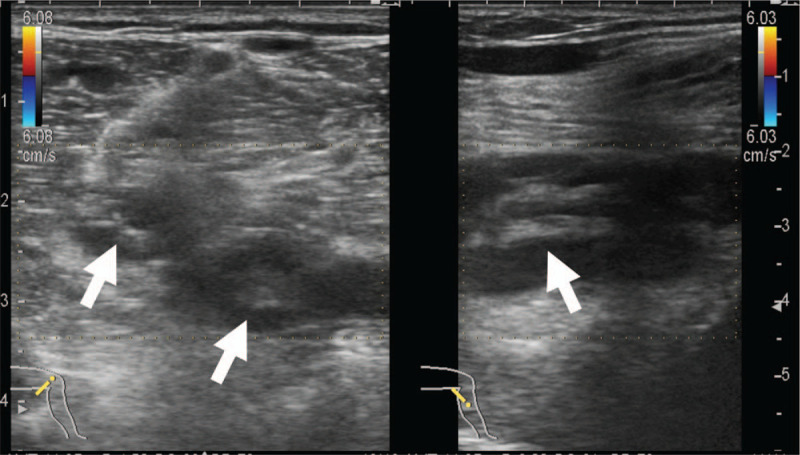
Venous ultrasonography showing an organized thrombus in the left femoral vein (arrows).
